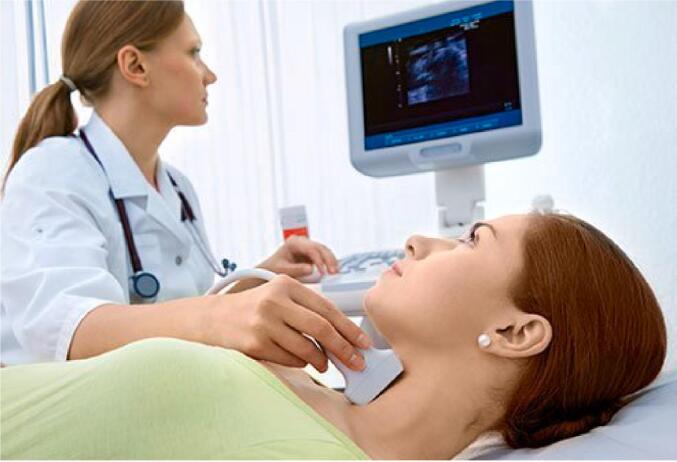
An ultrasonic scanning equipment for diagnosing thyroid cancer. Image courtesy of WebMed. ©2021, WebMD, LLC. All rights reserved[11].
